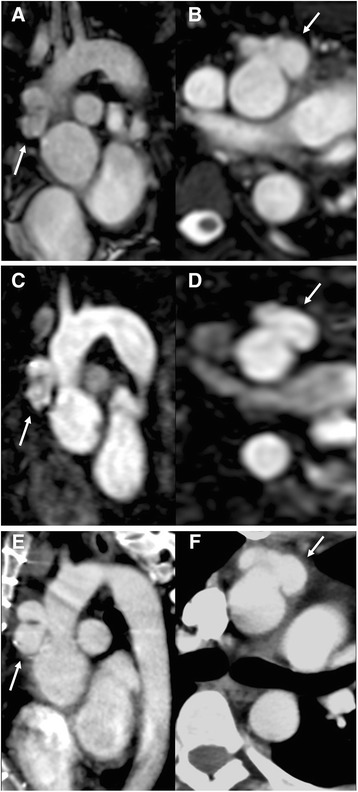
Postoperative suture dehiscence: Postoperative suture dehiscence with consecutive aneurysm at the distal aortic suture line 1-year after David procedure in a 20-year-old man with Marfan syndrome. Both (a, b) non-contrast 2D bSSFP and (c, d) 3D CE-CMRA demonstrate the aneurysm (arrows) with high image quality (4 points by both readers). The CMR-based diagnosis was confirmed with (e, f) computed tomography angiography. The patient underwent immediate surgical revision with re-replacement of the aortic root for treatment of his potentially life-threating complication.
